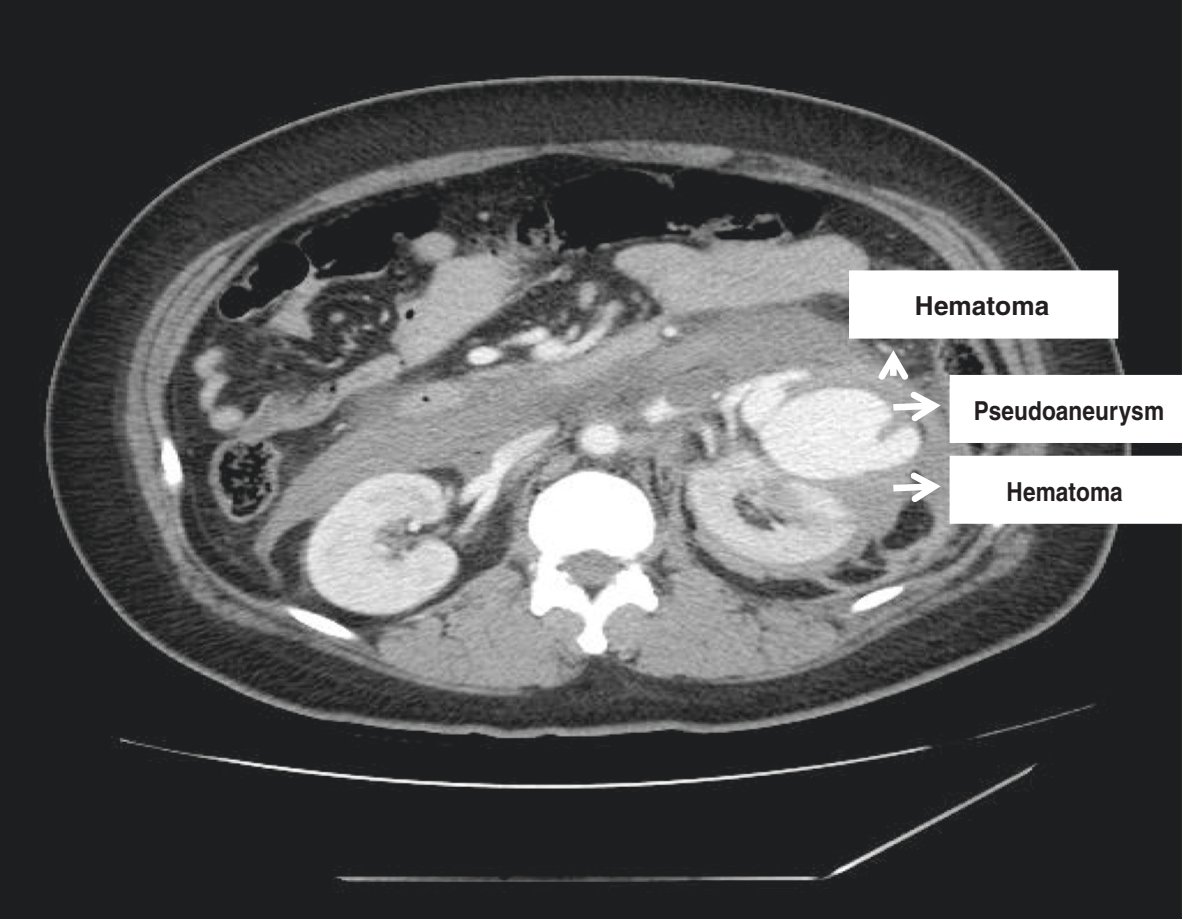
Computed tomography scan confirmed a large retroperitoneal hematoma from a left renal artery pseudoaneurysm, which extended through a gap in the anterior renal fascia from a left perirenal hematoma.
